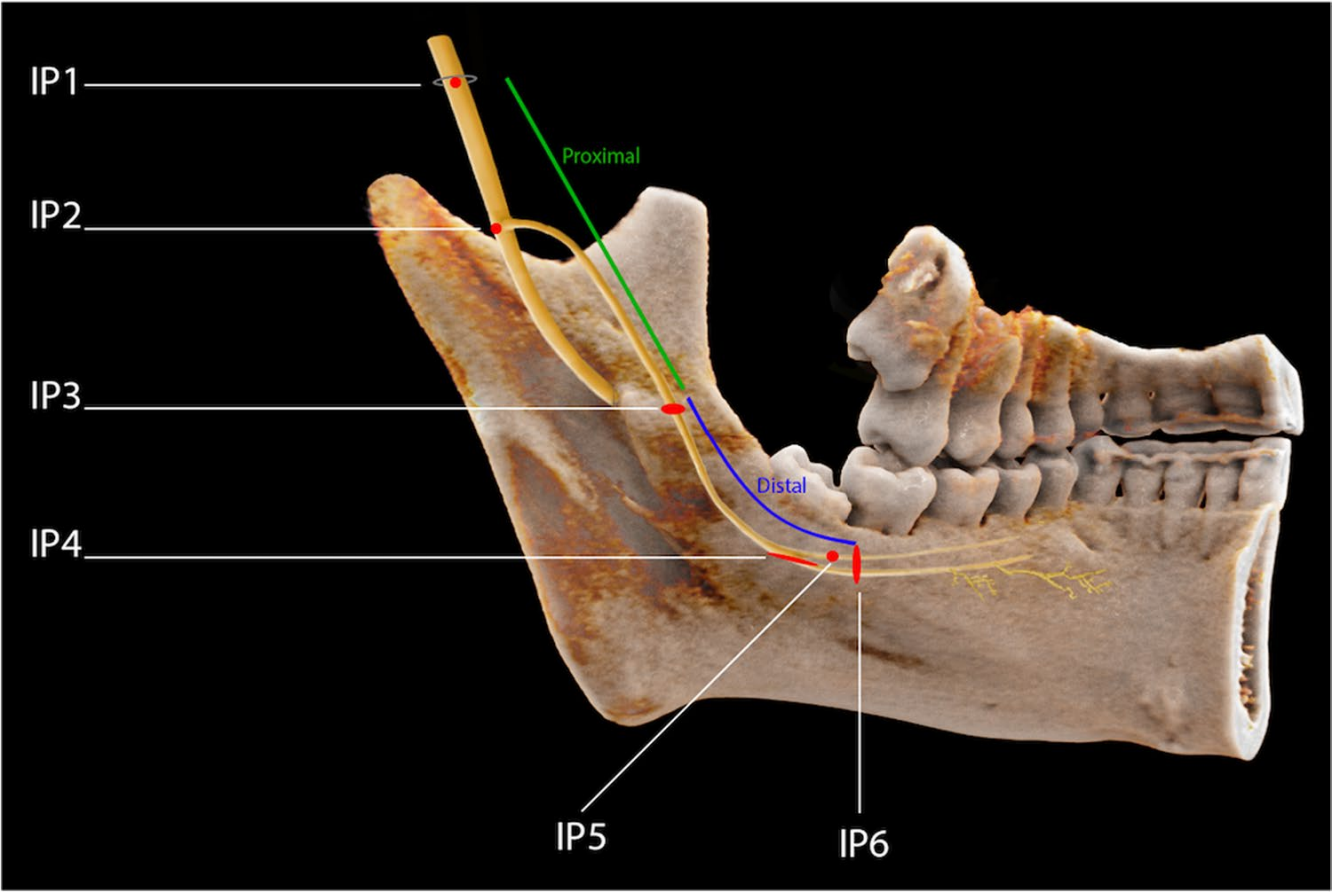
Photorealistic 3-dimensional (3D) visualization of a study participant’s cone beam computed tomography (CBCT) using cinematic rendering (CR). The division of the mandibular and lingual nerve (LN) into a proximal (IP1–IP3) and distal portion (IP3–IP6) with the 6 intermediate points (IP) is visualized. IP1, oval foramen; IP2, branching into the IAN and LN; IP3, LN starts running from laterally to medially; IP4, LN at the level of the third molar; IP5, gingival branch of the LN; IP6, LN at the height of the second molar
